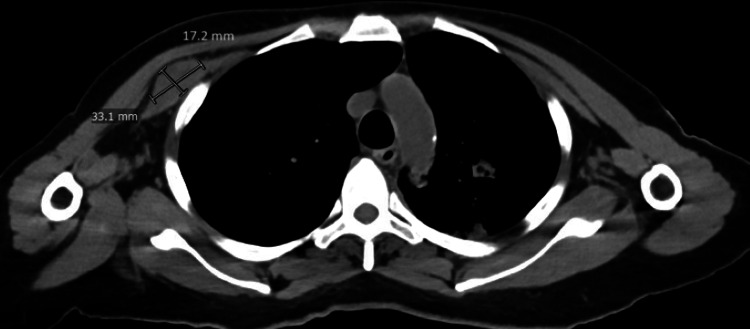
Chest CT - Right pectoralis minor mass measuring 33.1 mm x 17.2 mm
